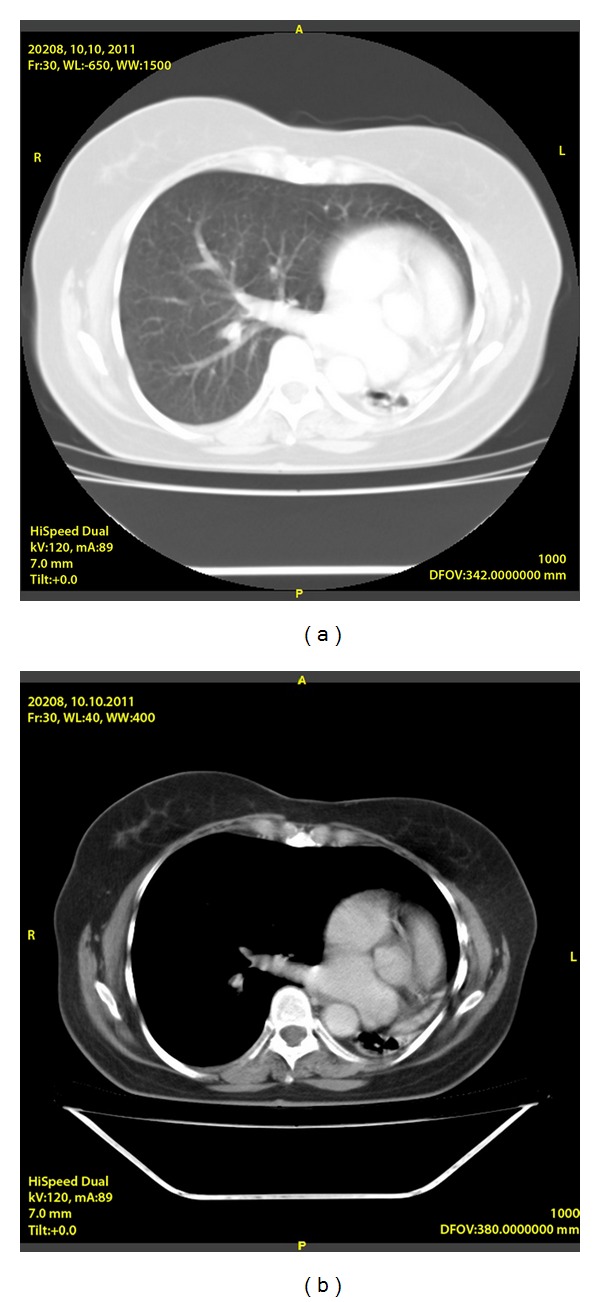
Thoracic CT scan revealing absence of left pulmonary artery and ipsilateral pulmonary hypoplasia.
